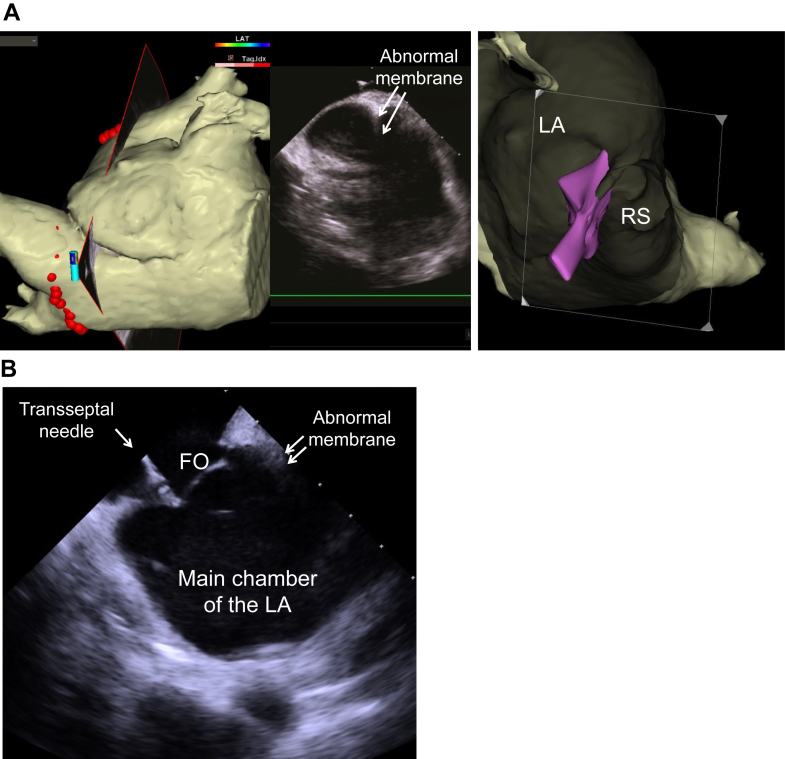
A: Intracardiac echocardiography shows the abnormal membrane subdividing the left atrium (LA) into 2 chambers and the CARTO merged image using the 3-dimensional computed tomography. B: Intracardiac echocardiography at the time of transseptal puncture. The septal-side edge of the membrane coincided with the upper edge of the fossa ovalis (FO). The entire FO faced the main chamber of the LA. RS = right superior pulmonary vein.
